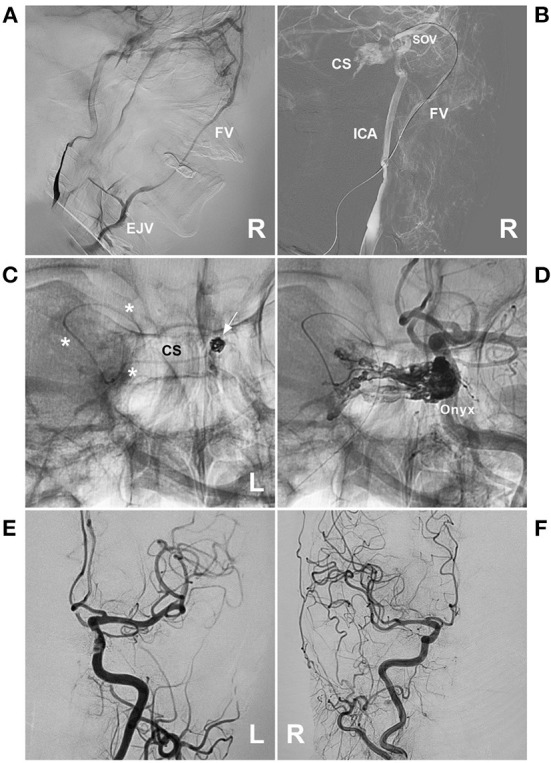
Operative angiography of CS-DAVF embolized *via* the trans-EJV-FV-SOV route. (A) Venous phase angiography of the right ICA shows the right FV into the EJV. (B) Venous navigation roadmap shows that the microcatheter passed through the right angular vein into the SOV. (C) X-ray image shows the microcatheter (asterisks) passing through the intercavernous sinus from the right into the left CS to perform coiling (arrow). (D) Unsubtracted angiography shows the combined use of Onyx to occlude the CS-DAVF. (E, F) Post-TVE angiographies of the left carotid artery (E) and right carotid artery (F) show complete occlusion of the DAVF. CS, cavernous sinus; DAVF, dural arteriovenous fistula; EJV, external jugular vein; FV, facial vein; ICA, internal carotid artery; L, left; R, right; SOV, superior ophthalmic vein; TVE, transvenous embolization.
